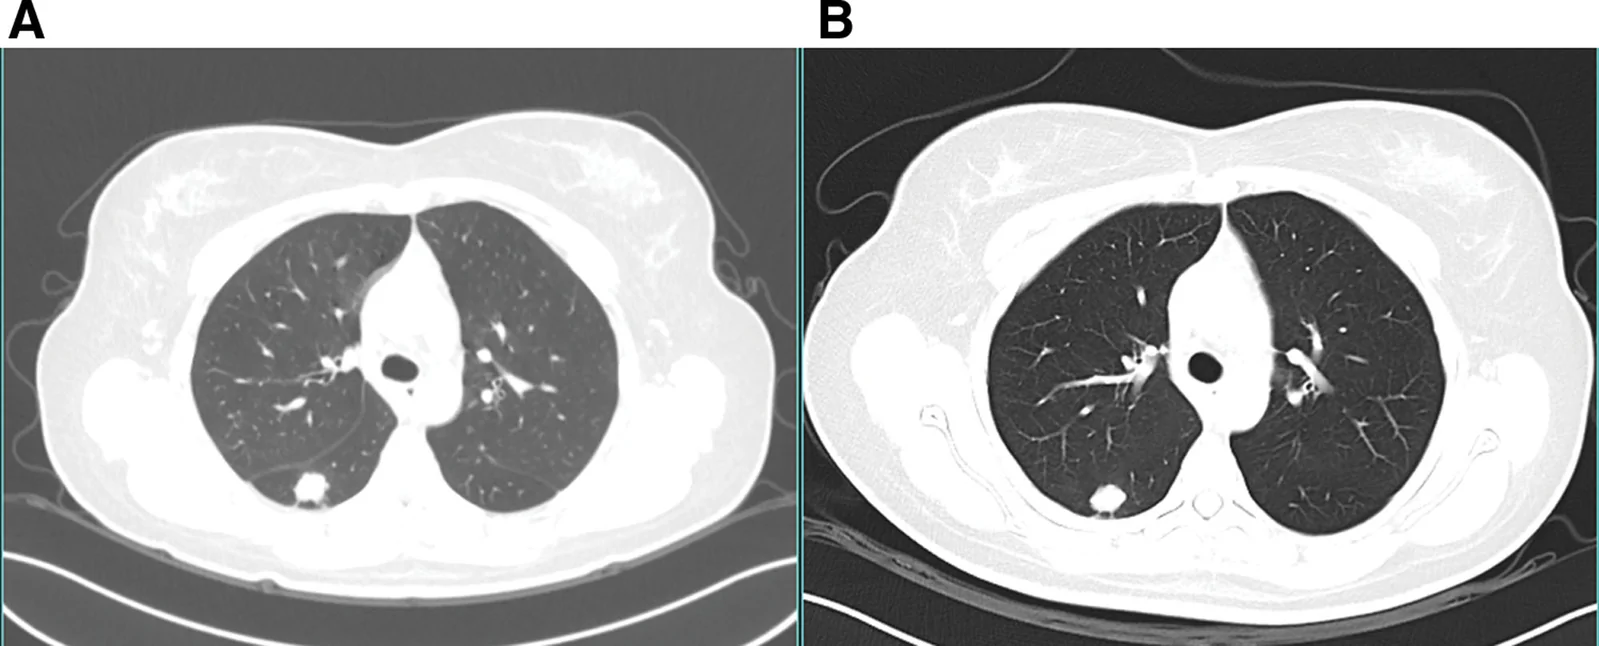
(A) A CT scan of the chest revealed a 1.7 cm × 1.5 cm solid nodule in the dorsal segment of the right lower lobe, with burrs and lobes on edge, and the adjacent pleura was stretched and thickened. (B) Contrast-enhanced CT scan showed heterogeneous enhancement. CT = computed tomography.
